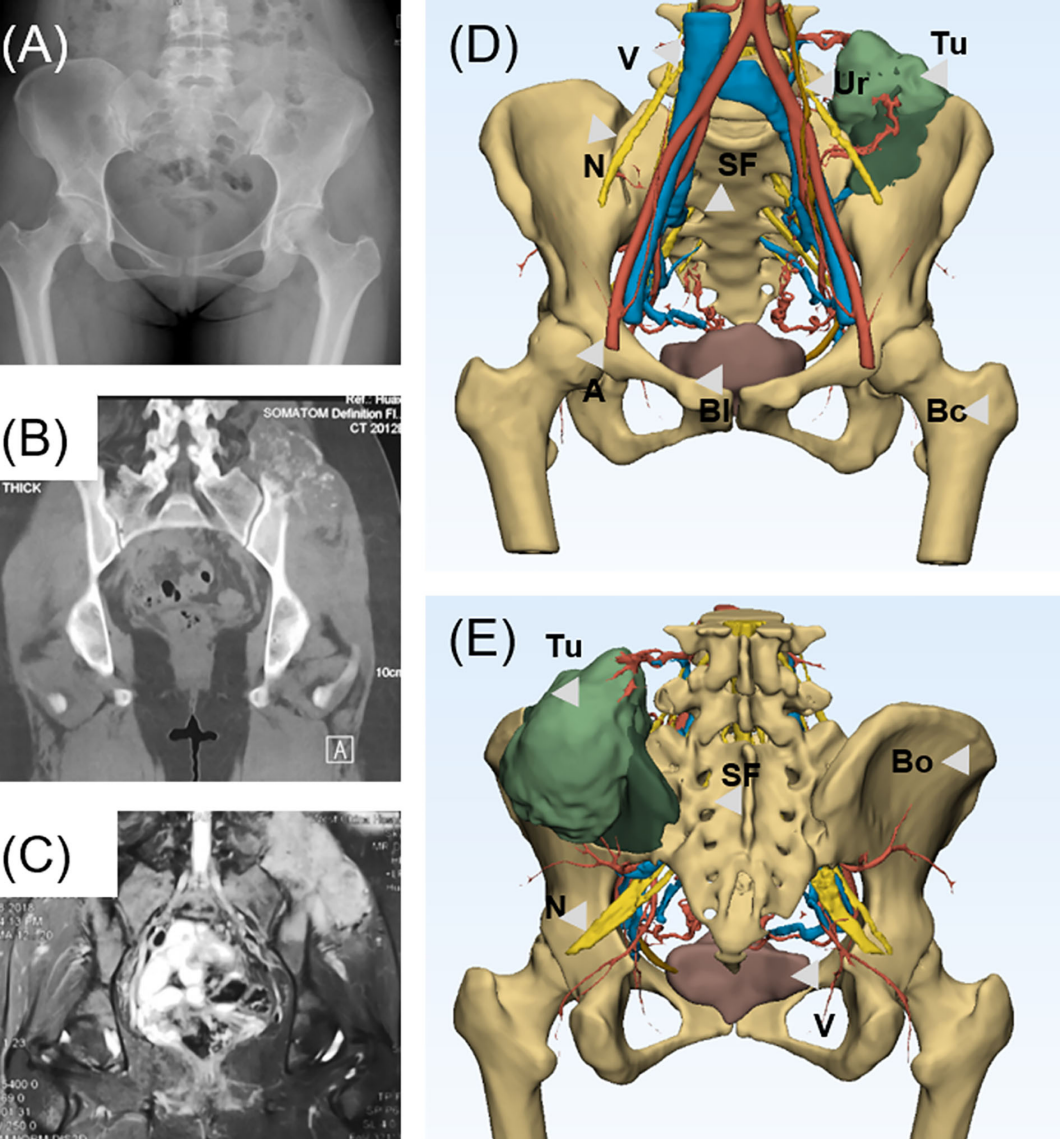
(A) Preoperative X-ray shows the bone destruction invading the left ilium and sacroiliac region. (B) Computed tomography shows the chondrogenic bone destruction in left sacroiliac region with soft tissue mass. (C) Magnetic resonance imaging shows an extensive soft tissue involvement invading sacroiliac region. (D) Anterior view of 3D-multimodality image of tumor model of various structures presented. (E) Posterior view of 3D-multimodality image of tumor model. 3DMMi, 3D-multimodality image; A, artery; Bl, bladder; Bo, bone; Bc, bone cortex; Ki, kidney; N, nerve; SF, sacral foramina; Tu, tumor; Ur, ureter; V, vein.
